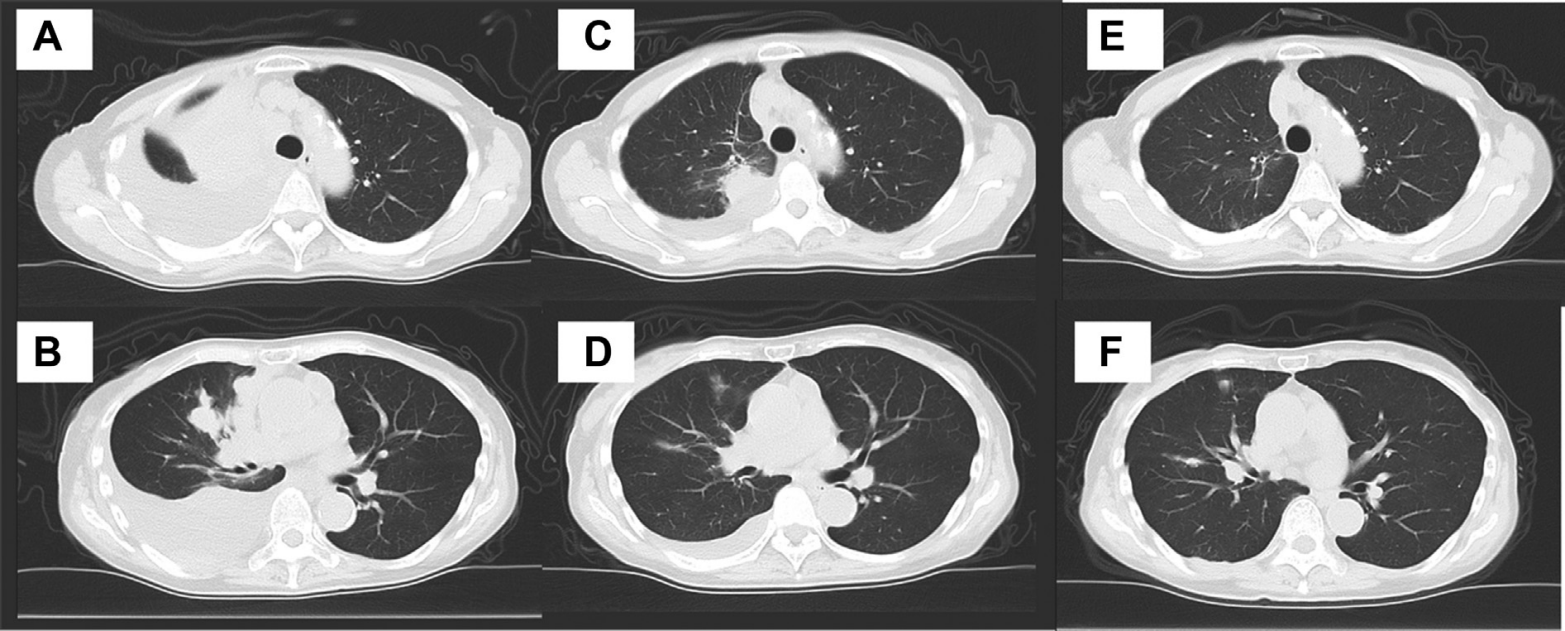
Clinical course based on CT before and after sotorasib. (*A* and *B*) Just before sotorasib administration. (*C* and *D*) One month after sotorasib administration, the tumors had shrunk and pleural effusion had decreased. (*E* and *F*) Four months after sotorasib administration, antitumor effects of sotorasib persist. CT, computed tomography.
